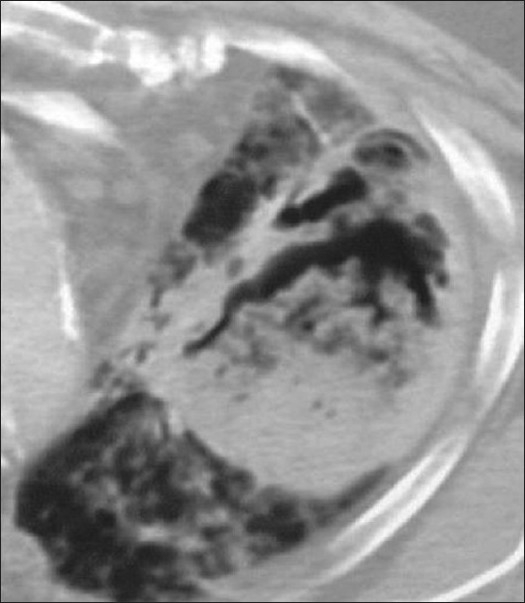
Aspergillosis. Angioinvasive disease chest X-ray is the most common, manifesting as thick-walled cavitary lesions predominating in the upper lobes, with air-crescents surrounding areas of desquamated infarcted lung. Here, we see all the described features on computed tomography
